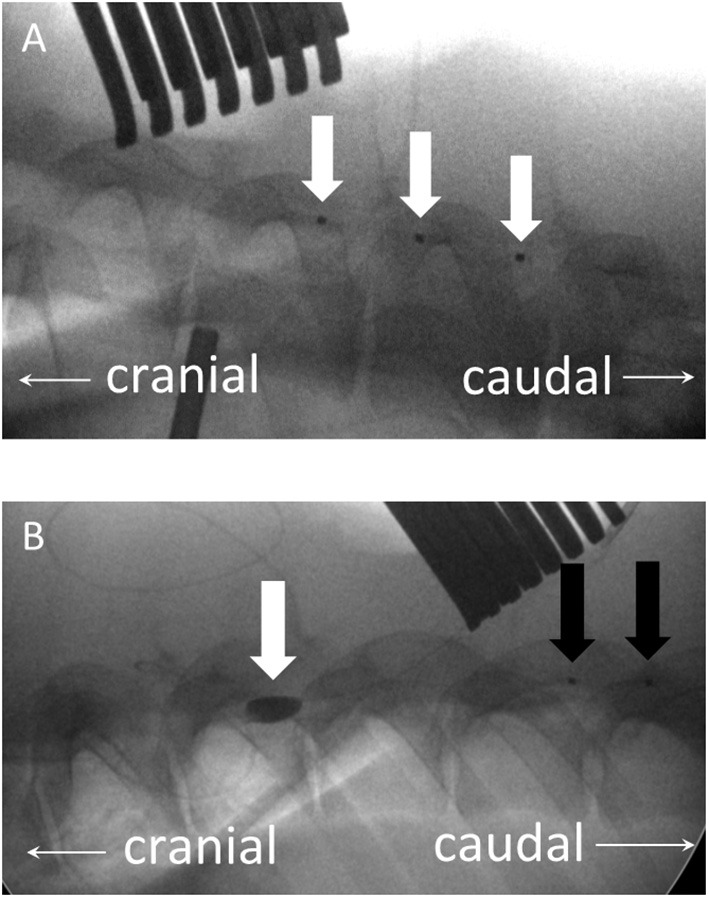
(A) Plain lateral radiograph showing an upstream three-contact platinum catheter electrode (D-wave electrode), inserted epidurally in the cephalic direction (white arrows) *via* a T5 flavectomy. (B) A downstream D-wave wire (black arrows) is inserted in the caudal direction epidurally from this laminectomy window. The balloon, which has been inflated with a contrast medium, is located at the T8 level (white arrow).
